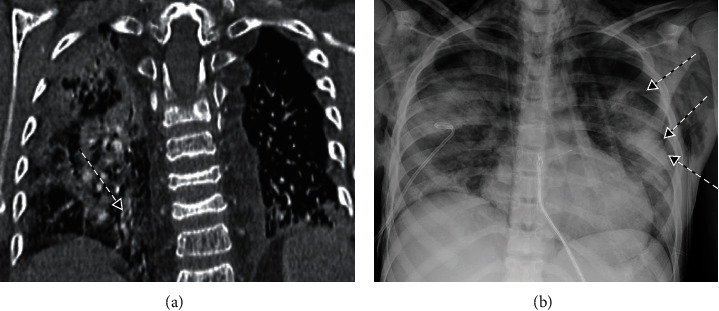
Variable COVID-19-related pathologies in immunocompromised patients: (a) chest CT angiogram demonstrating filling defects in the posterior and basal branches of pulmonary arteries, consistent with pulmonary embolism; (b) AP chest radiograph showing bilateral moderate to large pneumothoraxes.
